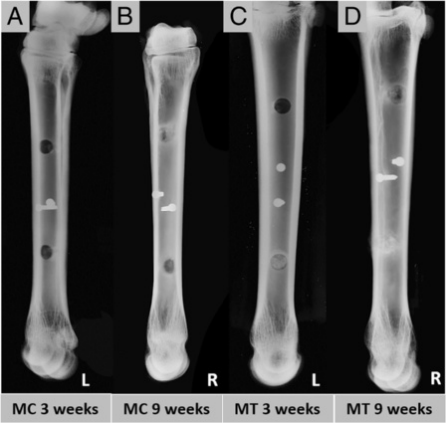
Radiographic faxitron images of the diaphyseal drill hole defects in the left and right metacarpal and metatarsal bone (a-d). Defects are located at the interface of the second to third quarter of each bone. For the exact sample harvesting after the observation time of either 3 or 9 weeks a 2 mm screw was placed in a distance of 2 cm to the drill hole (a-d). Image c shows the left MT bone in which the proximal drill hole was left empty and the distal drill hole was filled with autologous cancellous bone graft 3 weeks after surgery. 9 weeks after surgery the distal defect was hardly visible (autologous cancellous bone graft) (d) whereas the proximal empty control defect is still remarkable (c-d)
